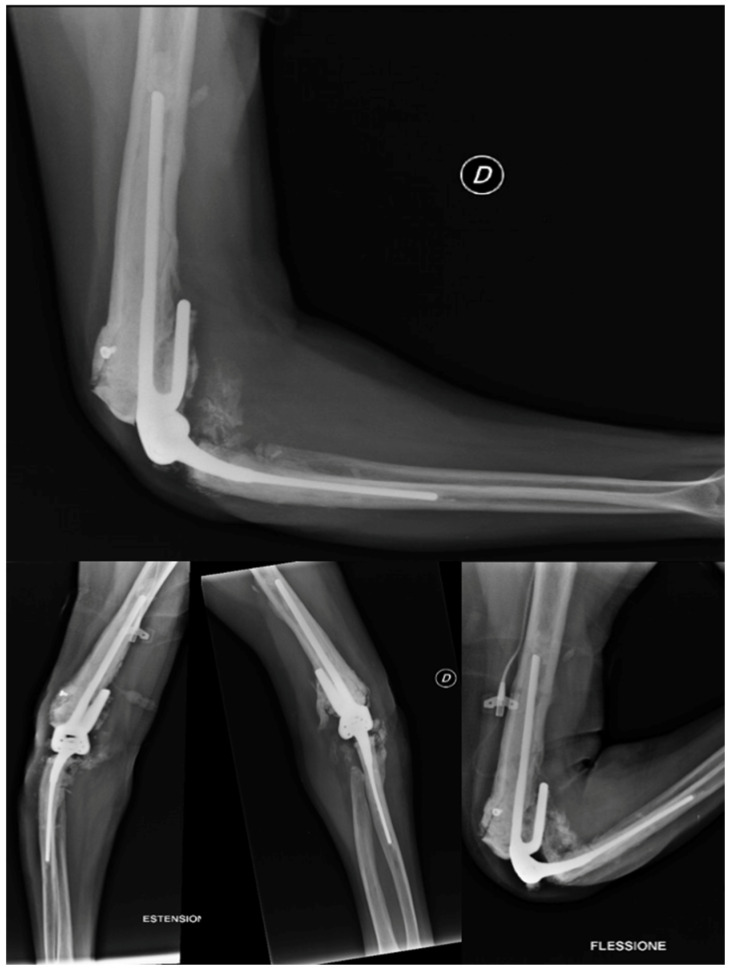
X-ray anteroposterior, lateral in neutral position, in extension and in flexion views of the right elbow at 6 months after the second revision surgery. No signs of loosening. Good alignment of the implant in neutral position and in flex–extension position.
